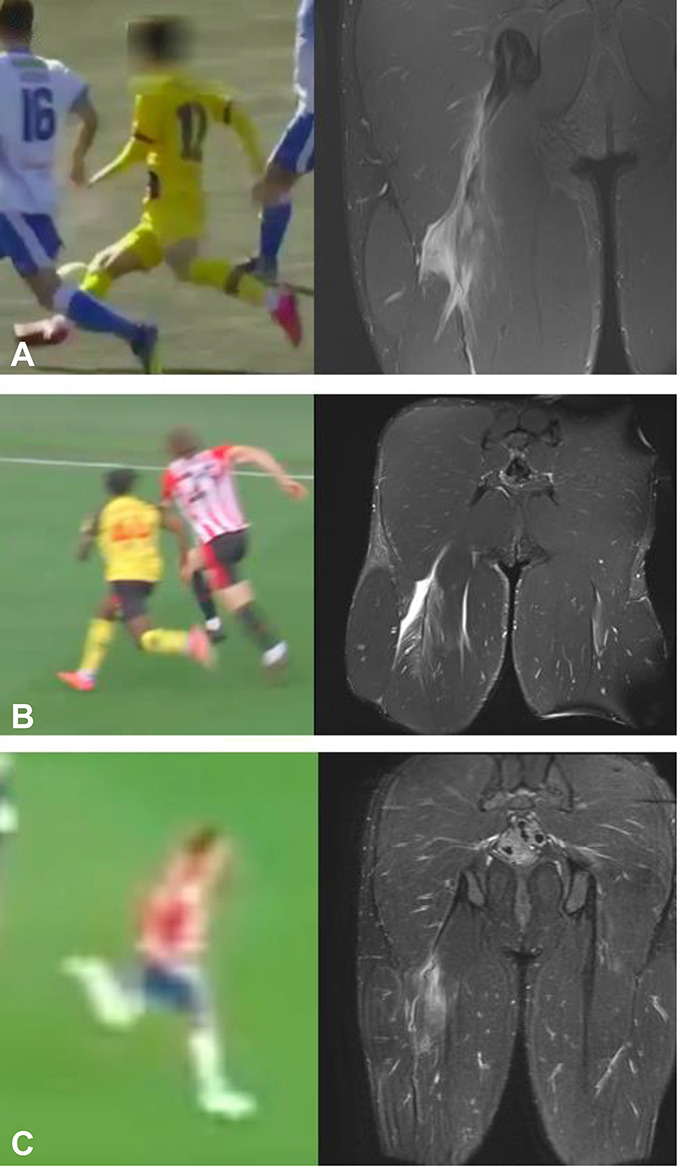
Assumed injury frames and MRI findings in sprint-type injuries. A, Case 4, proximal BF injury. B, Case 2, proximal BF injury. C, Case 3, proximal BF injury.
